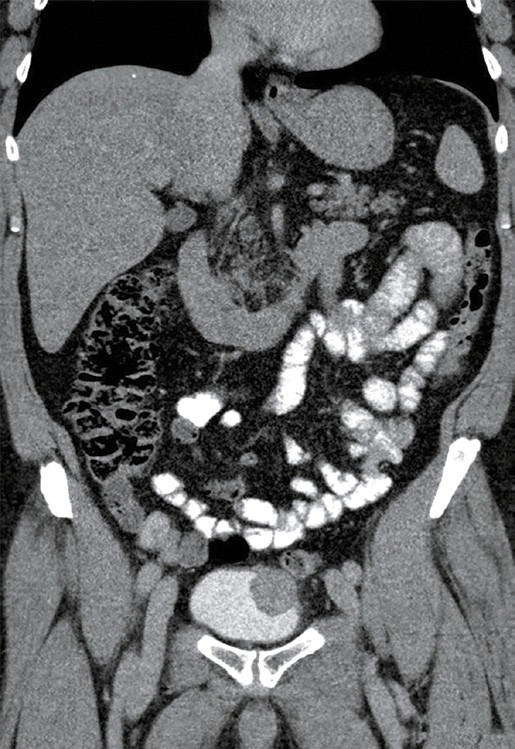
Computed tomography scan in the anteroposterior projection before surgery (tumor indicated by the arrow)
